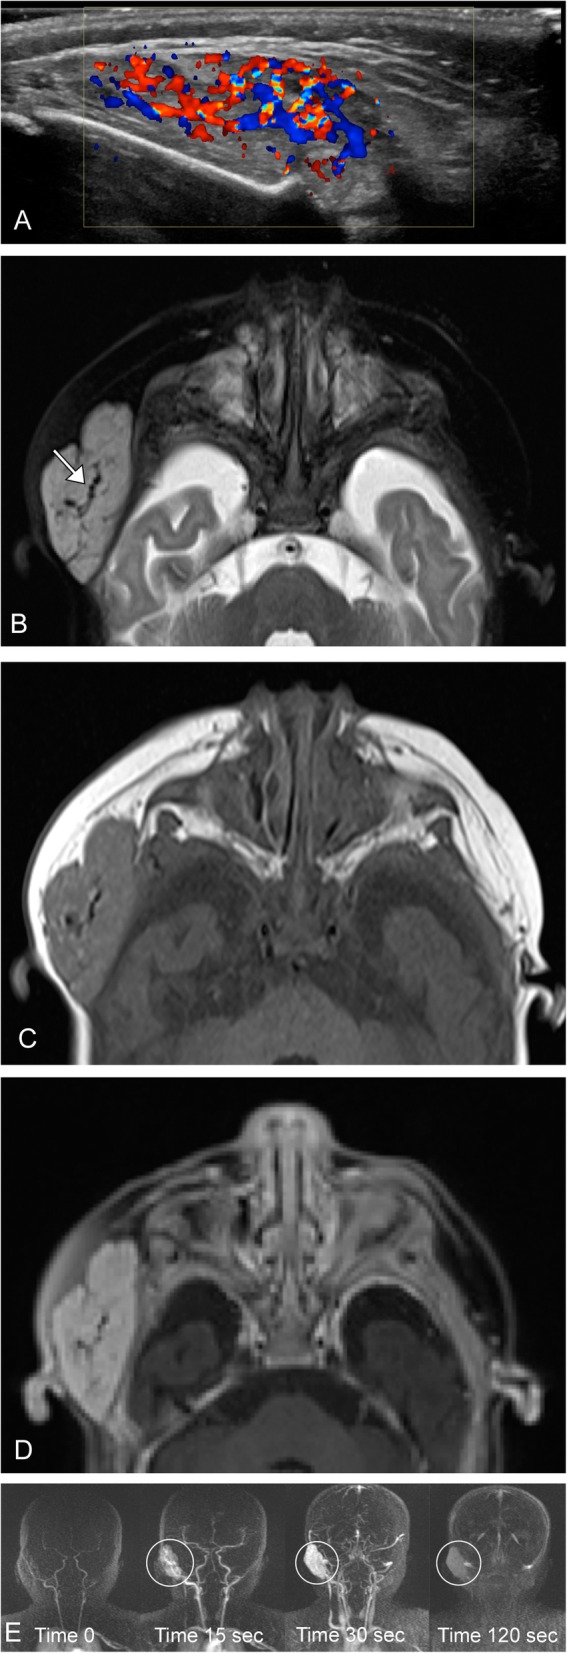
Infantile hemangioma. A-3 year-old female presents with a right pre-auricular face mass. a Ultrasound demonstrates a well circumscribed lesion at the area of concern with marked increased vascularity. b Axial T2 image demonstrates a mildly hyperintense mass (note: not as bright as cerebral spinal fluid) with lobulated borders and internal serpiginous vascular flow voids (arrow). c Axial T1 pre- and (d) post-contrast T1 fat suppressed images demonstrate avid enhancement of the lesion. e Time resolved contrast imaging demonstrates the classic wash in, followed by washout on delayed imaging (circled)
